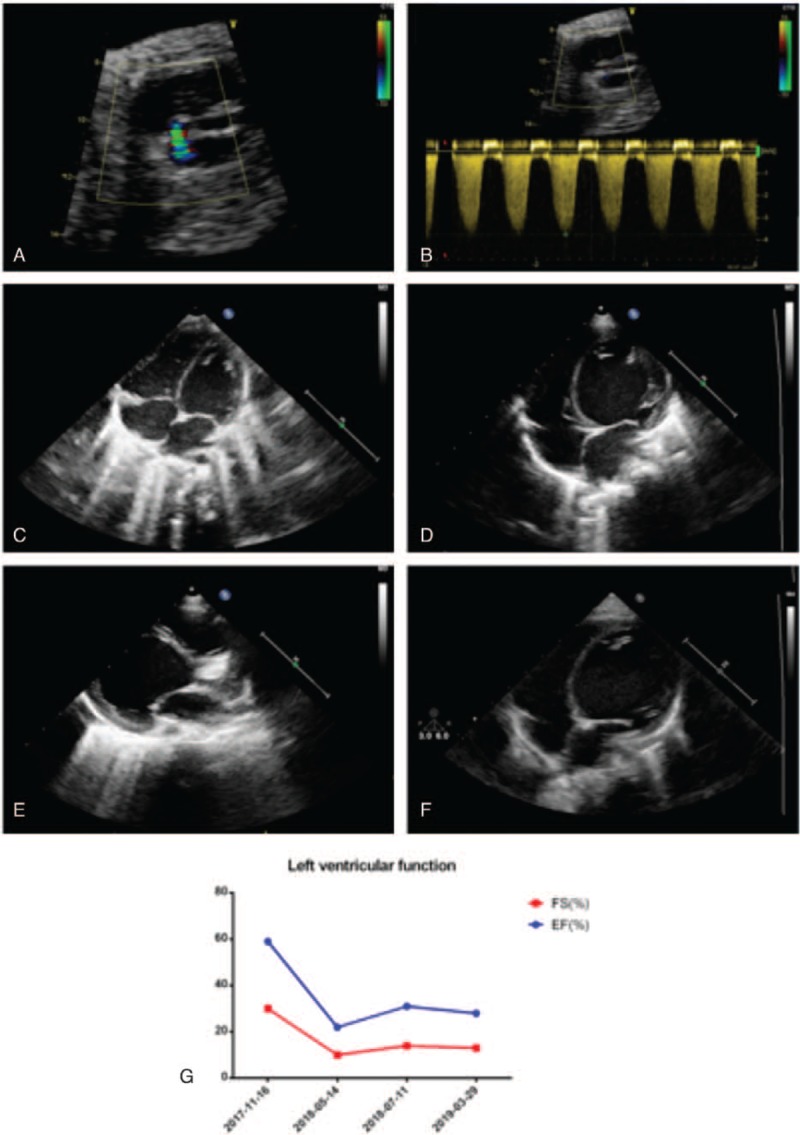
Echocardiography and the left ventricular function detection at each stage: (A-B) August 25, 2017, the fetal ultrasound showed severe tricuspid valve regurgitation, Vmax = 3.8m/s. 2D: LV = 11x19 mm, RV = 19 × 24 mm, LA = 12 × 10 mm, RA = 13 × 16 mm, AO = 5 mm, PA = 9 mm. Doppler: MV:E = 0.5 m/s, TV:E = 0.6 m/s, AV = 0.7 m/s, PV = 1.0 m/s; (C) November 16, 2017, the ultrasound showed that the size of each chamber was basically normal; (D-E) July 11, 2018, the ultrasound showed that the double ventricle was enlarged, with obvious left ventricle, slightly larger left atrium, and basically normal size of right atrium. (LV = 39 mm, RV = 15 mm, LA = 21 mm, RA = 26 mm); (F) March 29, 2019, the ultrasound showed that the left ventricle was significantly enlarged, and the size of the remaining ventricle was basically normal (LV = 40 mm RV = 8 mm LA = 18 mm RA = 28 mm); (G) the left ventricular function measurement followed the timeline.
